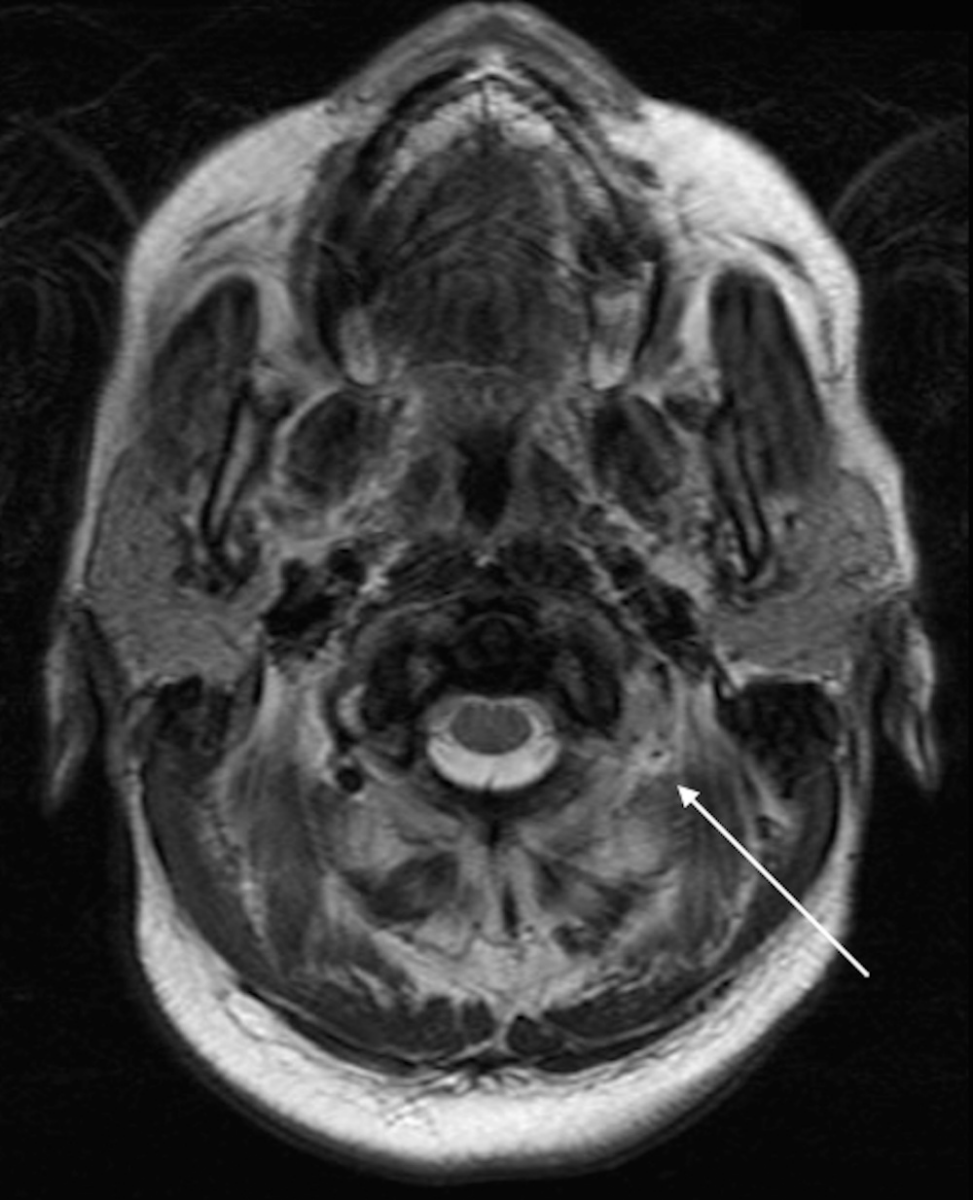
T2 magnetic resonance imaging (MRI) demonstrating flow void in the distal left vertebral artery (arrow) concerning for vertebral artery dissection.
